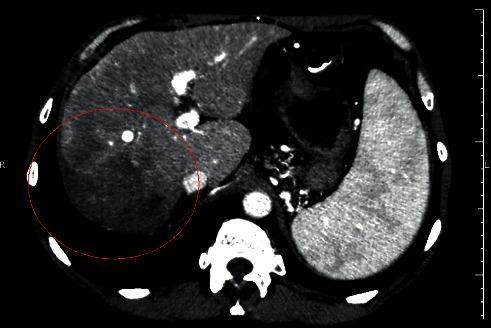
Computed tomography performed on April 8, 2019, showing a clear reduction of the liver tumor in the arterial phase, underscored by the red line. Instead of arterial phase enhancement, the tumor was now fulfilled by necrotic tissue.
